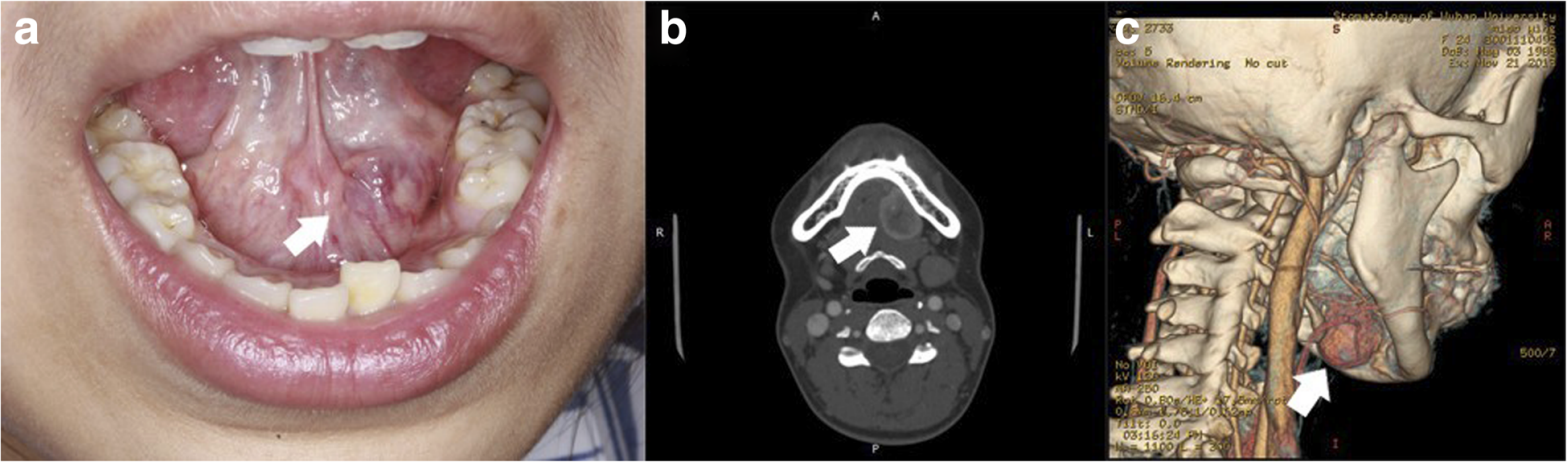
Physical examination and computed tomography findings. a A light bluish, solid neoplastic lesion measuring 3 cm in size was palpated in the left floor of the mouth, and the covered mucosa had no ulcers (arrow). b A computed tomography scan of the head and neck shows a well-defined, solid, heterogeneous enhanced nodule in the left floor of the mouth. There was no obvious infiltration in the surrounding soft tissue, and the lingual cortical bone of mandible was not involved (arrow). c A three-dimensional image showing the lesion was located lateral to the hyoid bone and adjacent to the left sublingual fossa of the mandible with abundant blood flow (arrow)
